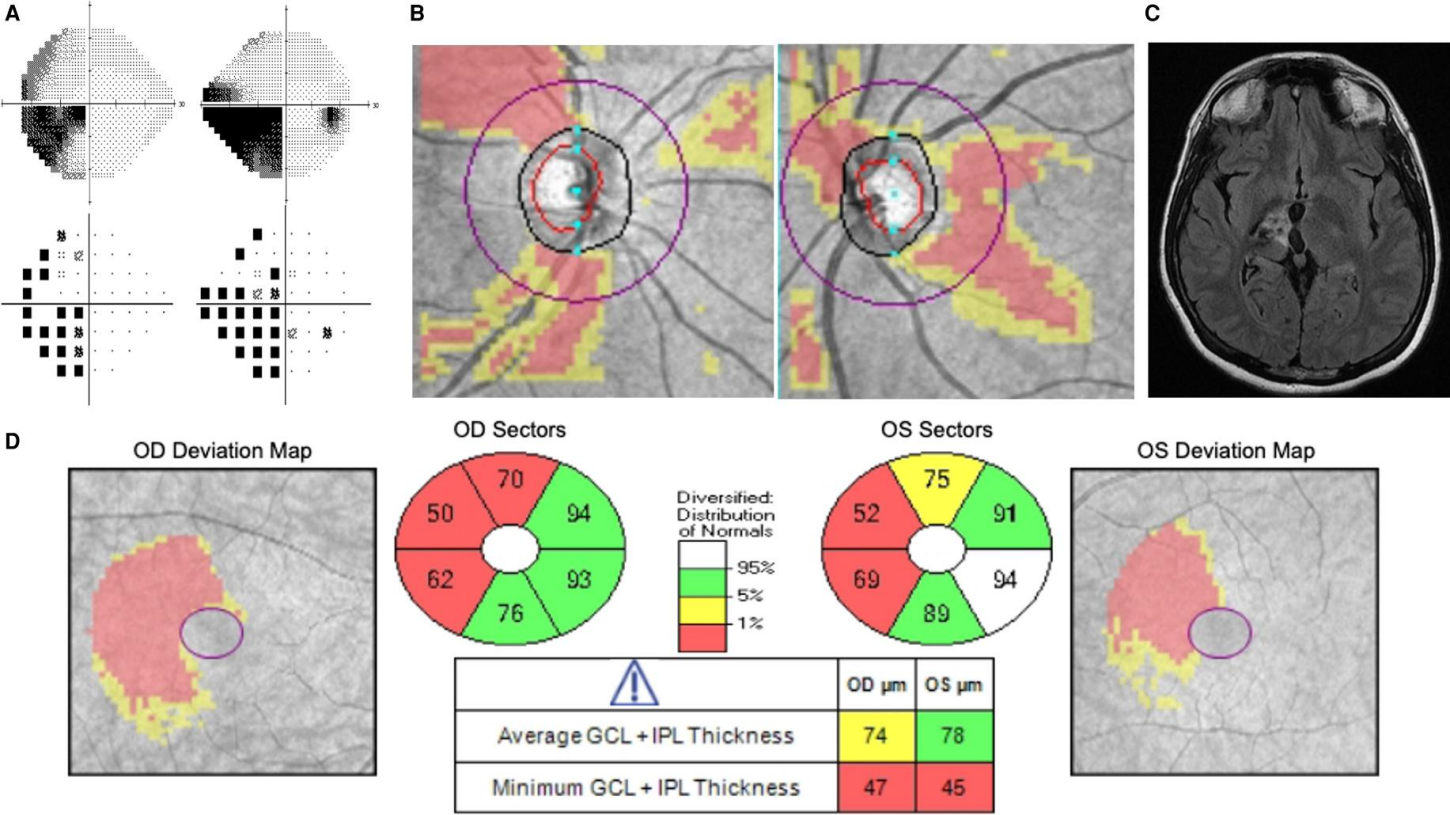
Optic tract syndrome. (A) Incomplete incongruous left HH seen on greyscale map and pattern deviation map, (B) hourglass atrophy of the right optic disc and band atrophy of the left optic disc, (C) post-radiation cystic changes following treatment of right thalamic arteriovenous malformation affecting the right optic tract (MRI axial FLAIR) and (D) right homonymous thinning of the GCL and inner plexiform layer (IPL) in both the right eye (OD) and the left eye (OS).
